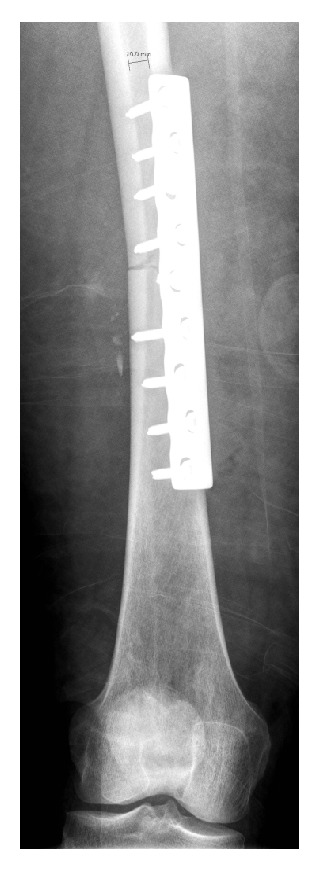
AP radiograph after temporary medial 4.5 mm LCP plate.
